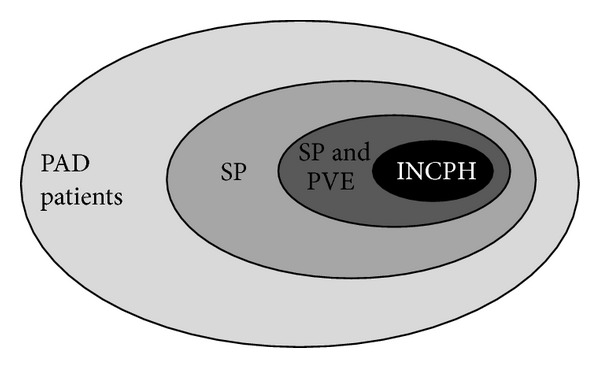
Distribution of PAD patients according to the abdominal ultrasound findings. PAD: primary antibody deficiencies; SP: PAD patients with isolated splenomegaly; SP and PVE: PAD patients with splenomegaly and portal vein enlargement; INCPH: PAD patients with idiopathic noncirrhotic portal hypertension.
